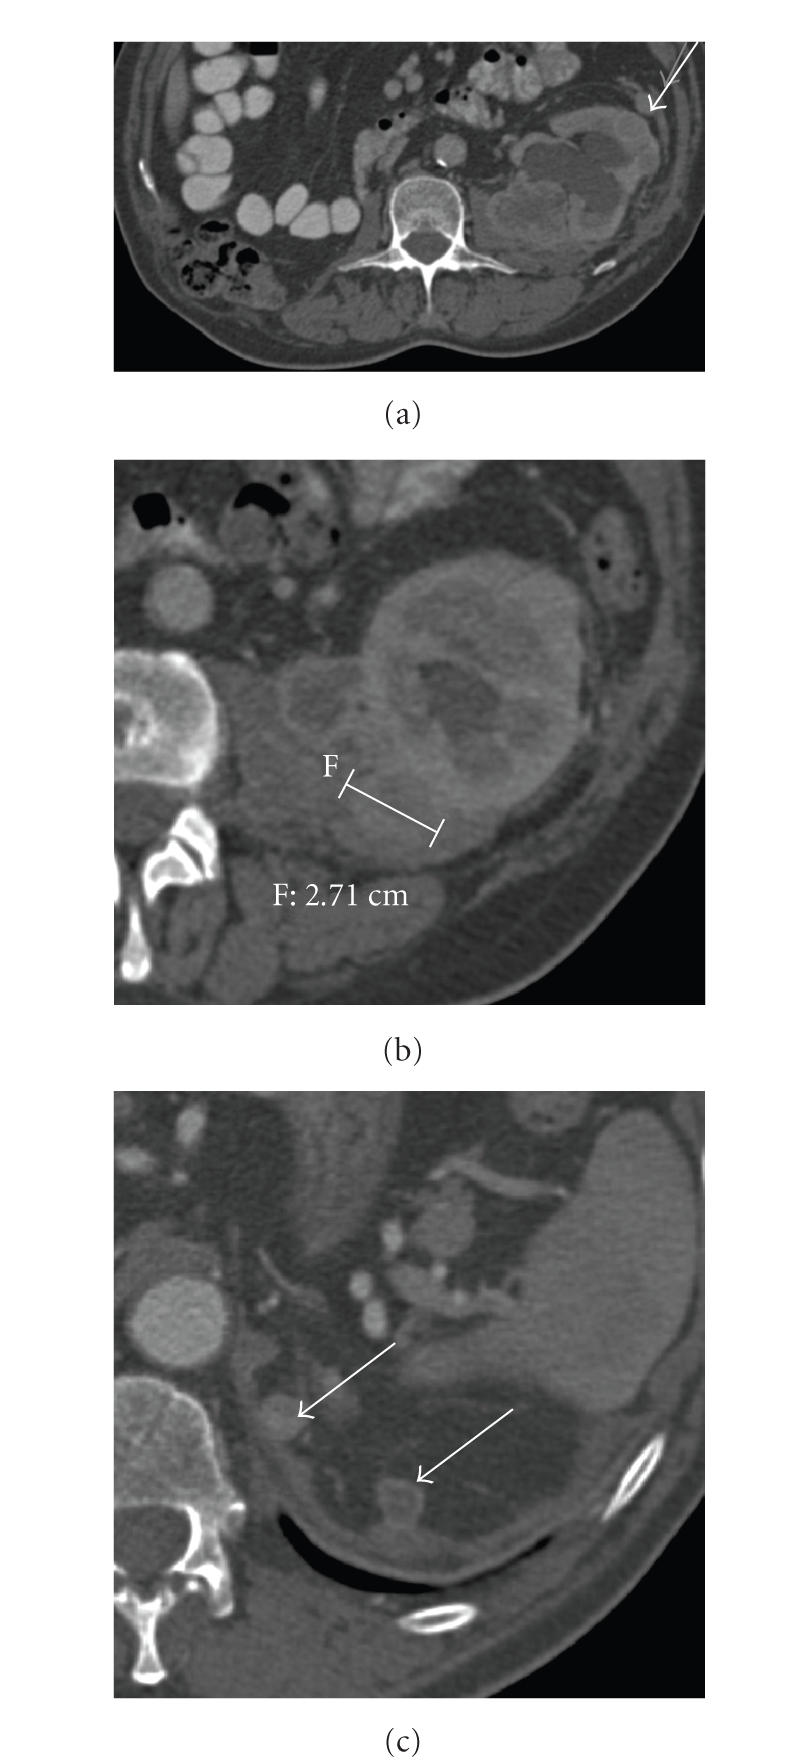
Local recurrence. (a) Axial contrast enhanced CT scan showing
